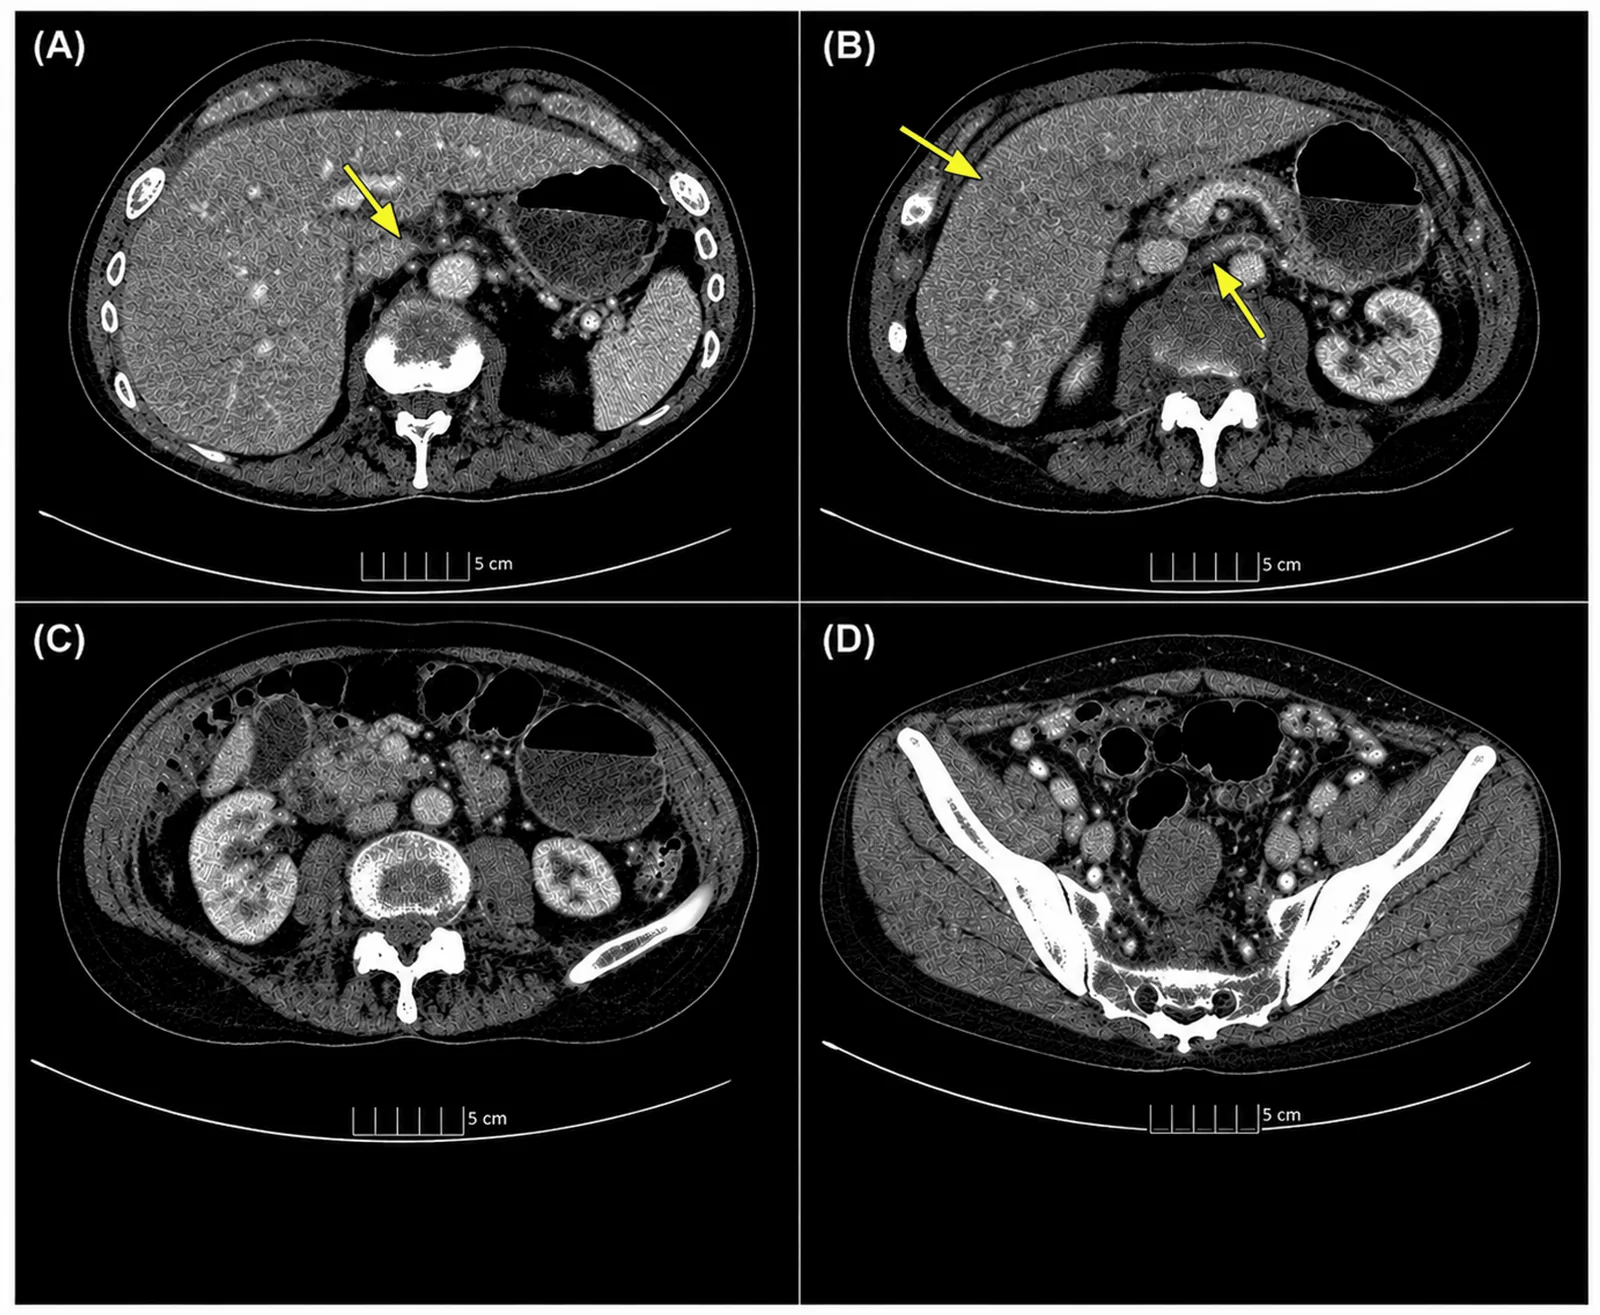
Contrast-enhanced CT of the abdomen and pelvis at initial presentation. (A, B) Axial contrast-enhanced CT images of the upper abdomen demonstrate mild inflammatory fat stranding surrounding the pancreatic head (arrow), with subtle periportal oedema within the left hepatic lobe (arrow). (C) Axial image through the lower abdomen demonstrates no additional acute intra-abdominal abnormality. (D) Axial pelvic image demonstrates no pelvic lymphadenopathy. There is no hepatomegaly, splenomegaly, biliary dilatation, radiopaque gallstones, or radiological evidence of acute cholecystitis.
